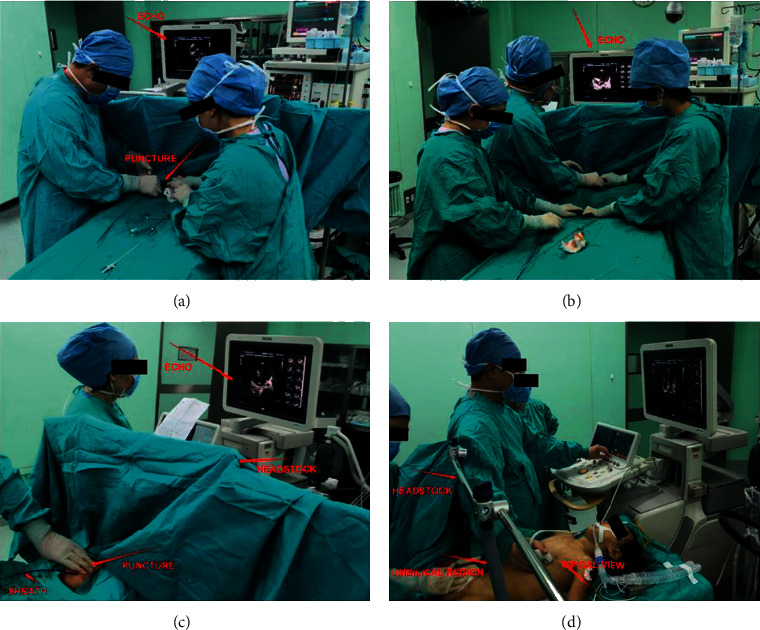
Percutaneous device closure of pediatric atrial septal defect through the femoral vein guided by transthoracic echocardiography without radiation: (a) in the operating room without radiological equipment, right femoral vein puncture point; (b, c) procedure under the guidance of transthoracic ultrasound; (d) the headstock (red arrow) and the child's umbilical region (red arrow) are vertically parallel, which can help isolate the surgical area and the ultrasound area.
